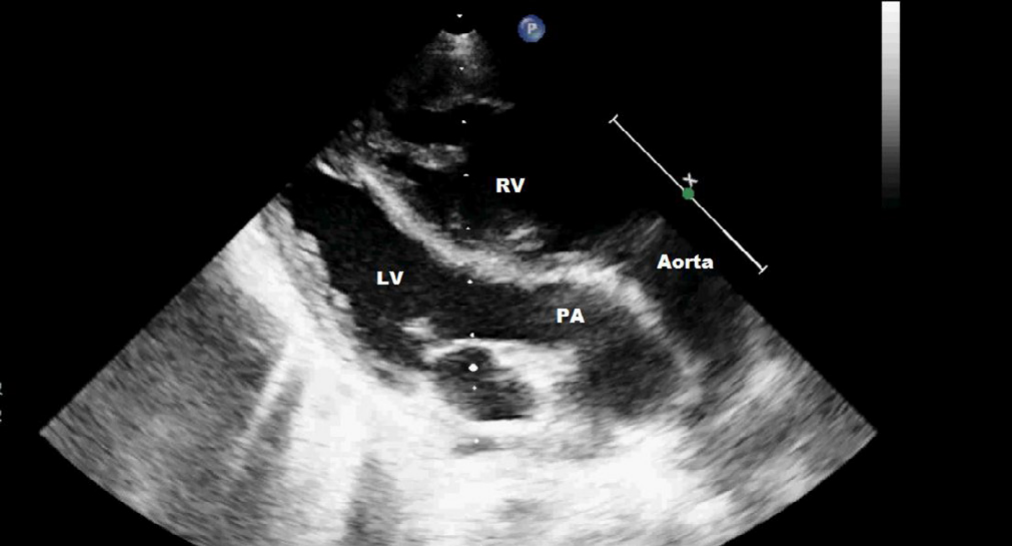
Parasternal long axis transthoracic echocardiography. LV=left ventricle; PA=pulmonary artery; RV=right ventricle
